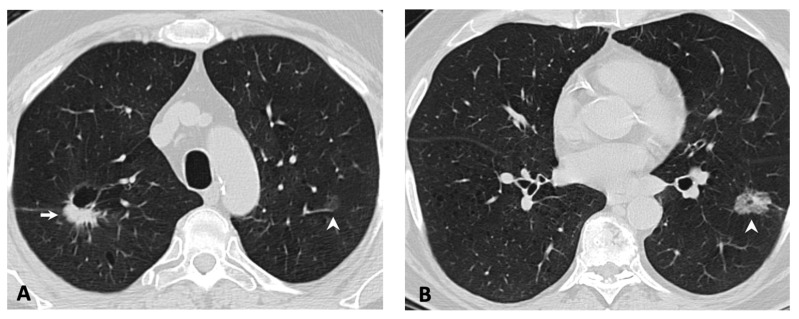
Multifocal lung adenocarcinomas in an 80-year-old female former smoker. (A) Axial CT through the upper lobes shows a part-cystic, part-solid nodule in the right upper lobe (A, arrow) and a faint ground-glass nodule in the left upper lobe (A, arrowhead). (B) Axial CT slice through the lower lobes shows an additional ground-glass nodule in the left lower lobe (B, arrowhead). Findings are consistent with multifocal adenocarcinomas. The patient went on to have a right upper lobectomy. Pathology and molecular testing revealed an adenocarcinoma with *MET*ex14 skipping mutation.
